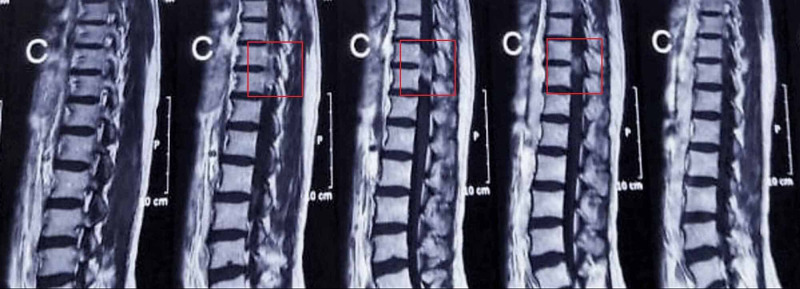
Magnetic resonance imaging scan (sagittal plane) of the thoracolumbar spine. The red boxes are showing the hyperintense lesion at level of T10-T11 on T1-weighted MRI.
